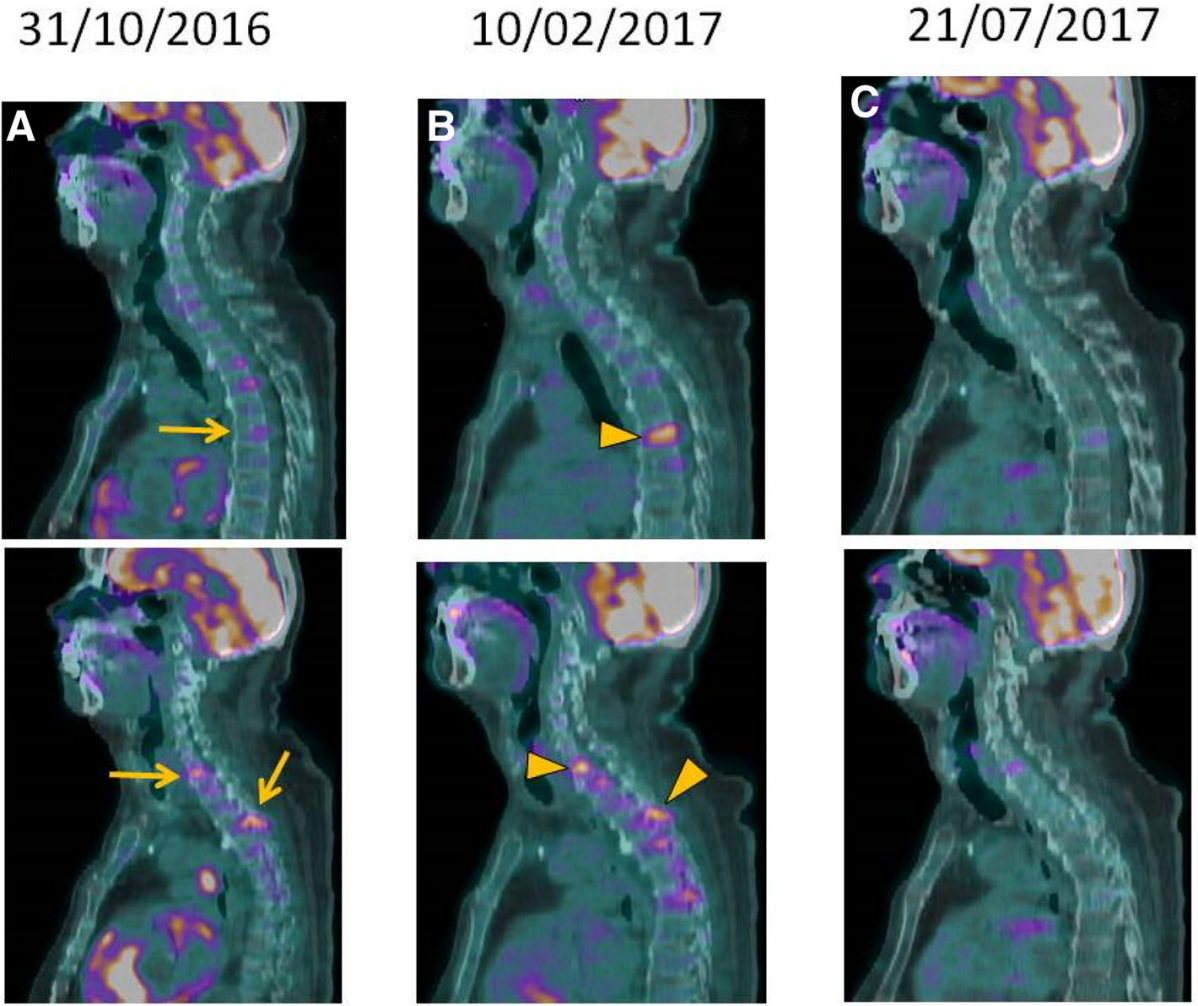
Bone Pseudoprogression after anti-PD1 therapy seen in ^18^F-FDG PET/CT’s. Sagital fused images from different ^18^F-FDG PET/CT’s. a Multiple focal FDG uptake in different thoracic vertebrae (T1, T3 and T7) without tomographic focal lesion (arrows), corresponding to bone metastasis. b Same corresponding thoracic bone lesions with significant increased uptake (arrowsheads), consistent with metabolic progression disease. c Metabolic resolution of most thoracic bone lesions, consistent with partial metabolic response (PMR)
